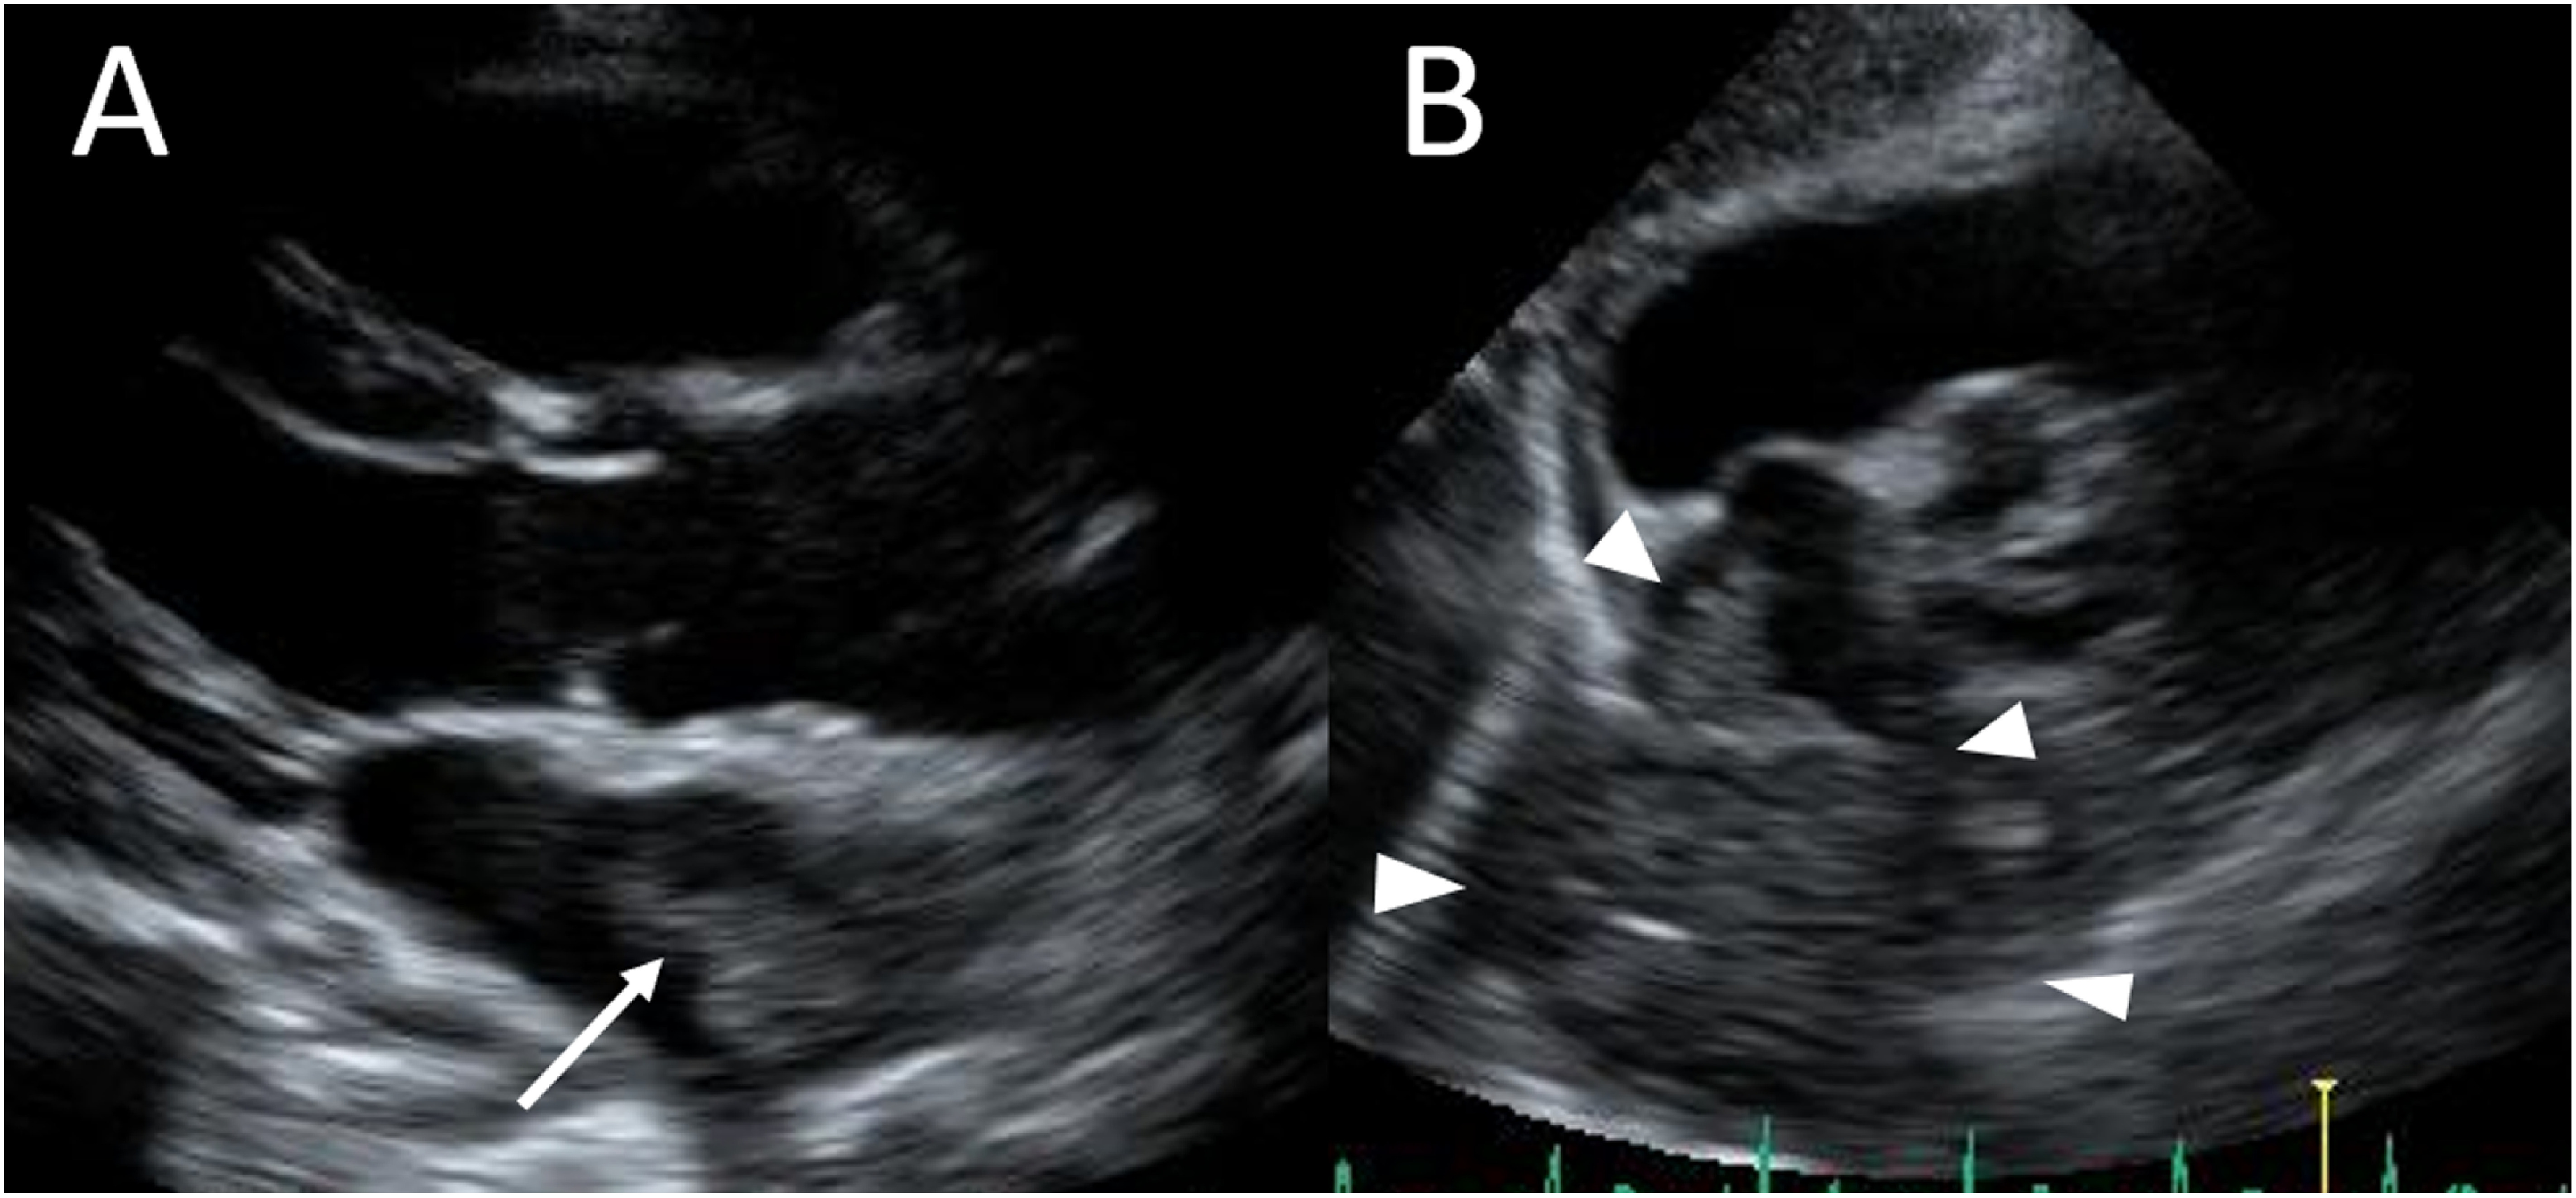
Fig. 2 Echocardiographic findings. (A) There was a highly mobile mass, as indicated by the arrow, in the left atrium (LA). (B) The mass in the LA had continuity with the tumor in the right main bronchus, as suggested by the arrowheads.
